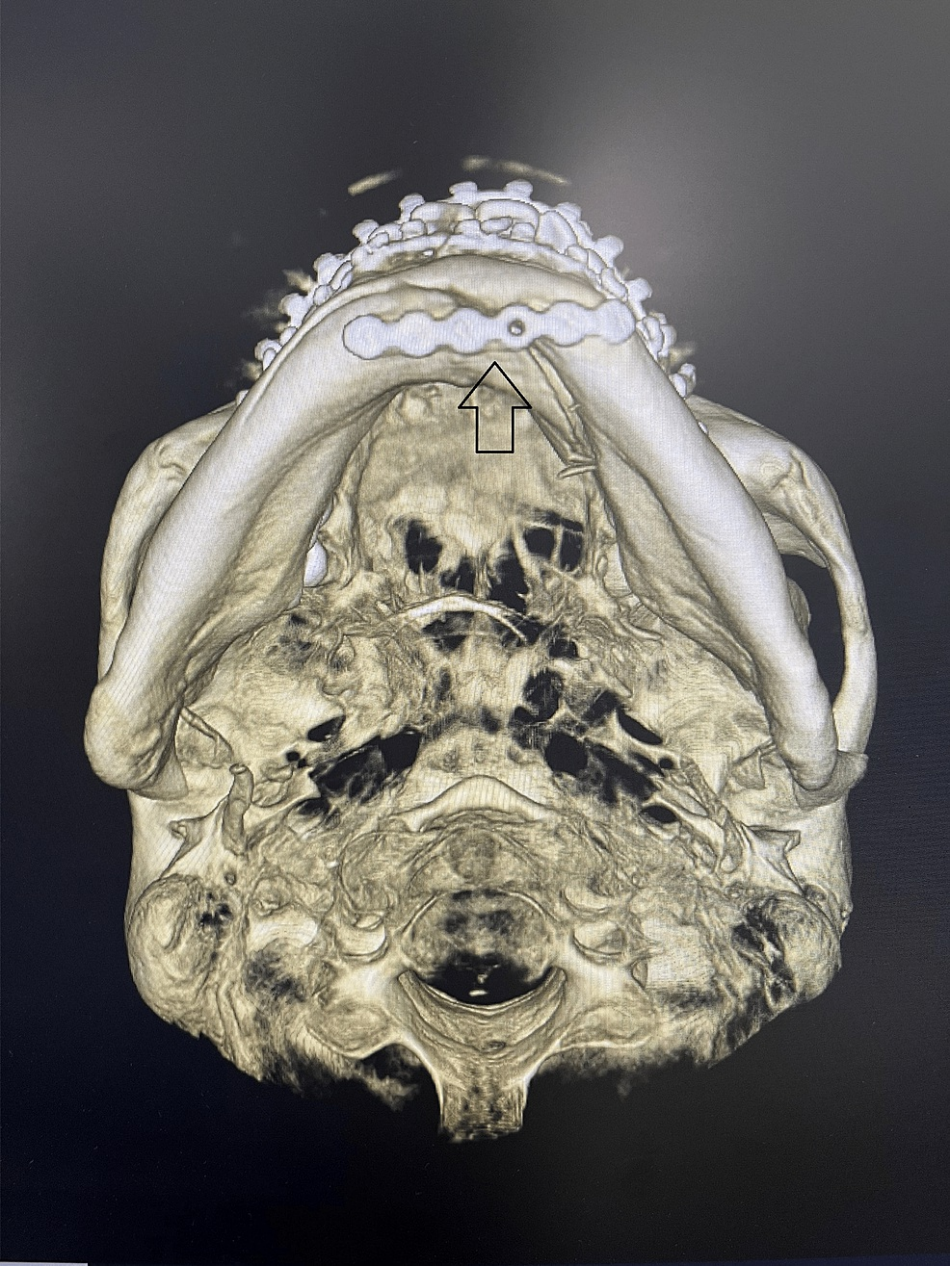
Post-operative radiograph (axial view) Post-operative radiograph depicting the reduced fracture and fixation using one 2mm five-hole plate secured using 2x8mm screws on the lower border of the mandible.
